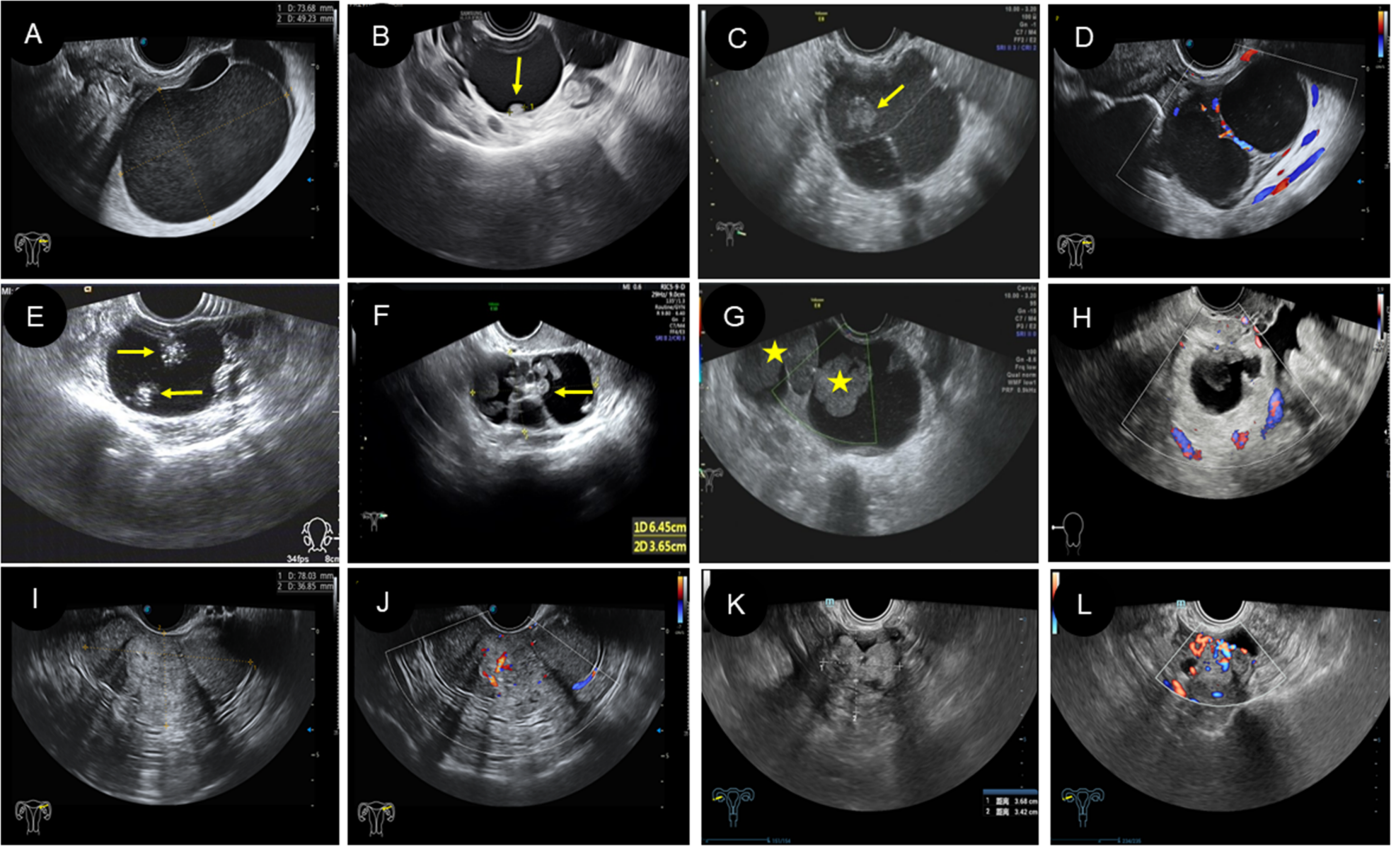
Two-dimensional and color Doppler ultrasound manifestations of different types of struma ovarii. (A–D) The ultrasound manifestations of cystic type of SO. (A) Two-dimensional ultrasound showed a large cyst with regular morphology and clear boundary, and the cyst is filled with weak echoes. (B) Two-dimensional ultrasound revealed a unilocular cyst with a papillary protrusion (arrow) in the cystic wall. (C) Two-dimensional ultrasound revealed multilocular cyst with several septations and “amniotic fat ball” (arrow) within the cyst. (D) On color Doppler flow imaging, blood flow signals were visible in the cystic wall and septa. (E–H) The ultrasound features of cystic-solid-type SO. (E, F) Two-dimensional ultrasound showed cystic-solid SO with several hyperechoic and different-sized nodules (arrow) in the cystic area (known as “strum pearls”). In addition, thick septa can be found in panel (F, G) The “amniotic fat ball” (asterisk) also can be found in the cystic area of cystic-solid SO patients. (H) On CDFI, blood flow signals were visible in the solid component. (I–L) The ultrasound characteristics of solid type of SO. (I, J) A solid-type SO patient showed regular morphology with rich blood flow. (K, L) A solid-type SO patient showed irregular morphology with rich blood flow. SO, struma ovarii; CDFI, color Doppler flow imaging.
